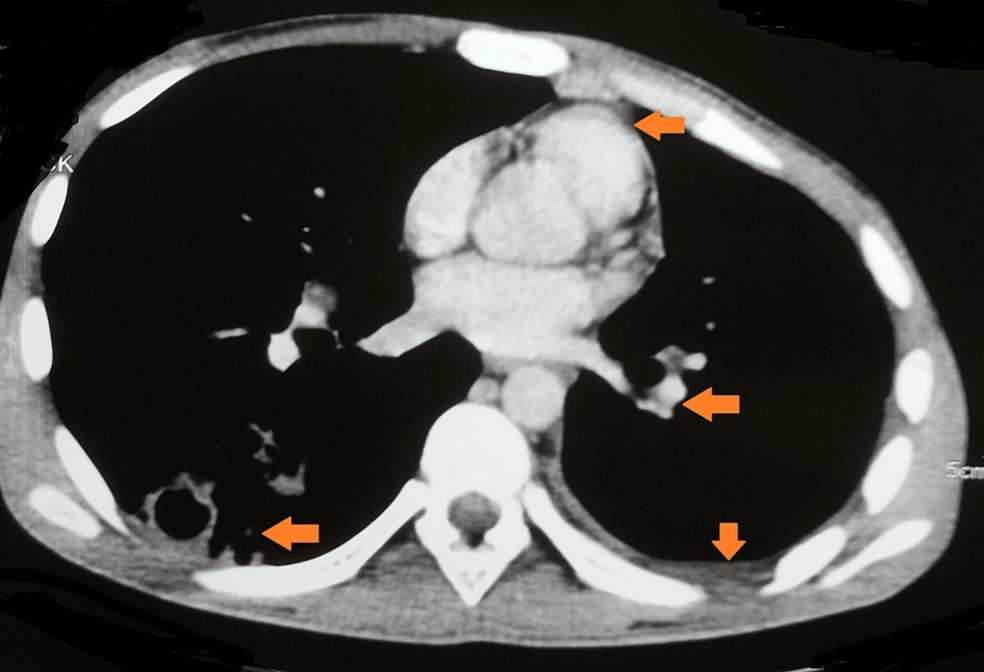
CT chest suggestive of pleural effusion, pericardial effusion, mediastinal lymphadenopathy, and pleural cavities with consolidation CT: computed tomography
